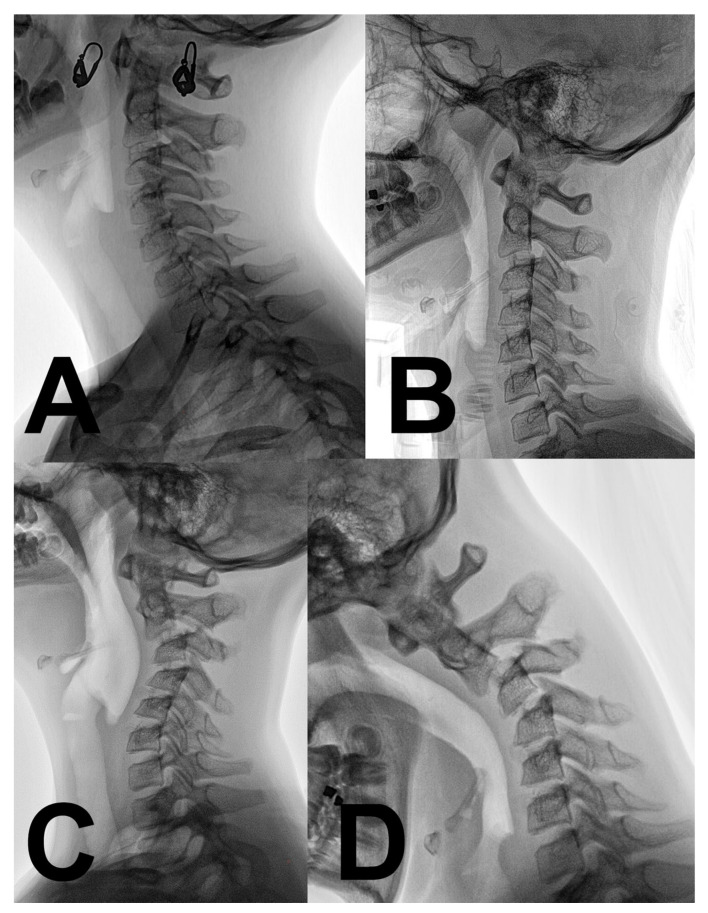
Radiographical evolution of progressive cervical kyphosis after a minor hyperflexion injury. (A) Physiological cervical lordosis immediately after a motor vehicle accident. (B) Loss of cervical lordosis and C2–3 pseudoluxation one week after trauma (hard collar fixation). (C) Cervical kyphosis progression 3 months after trauma. (D) Chin-on-chest relief position the deformity 6 months after the trauma, before corrective surgery.
